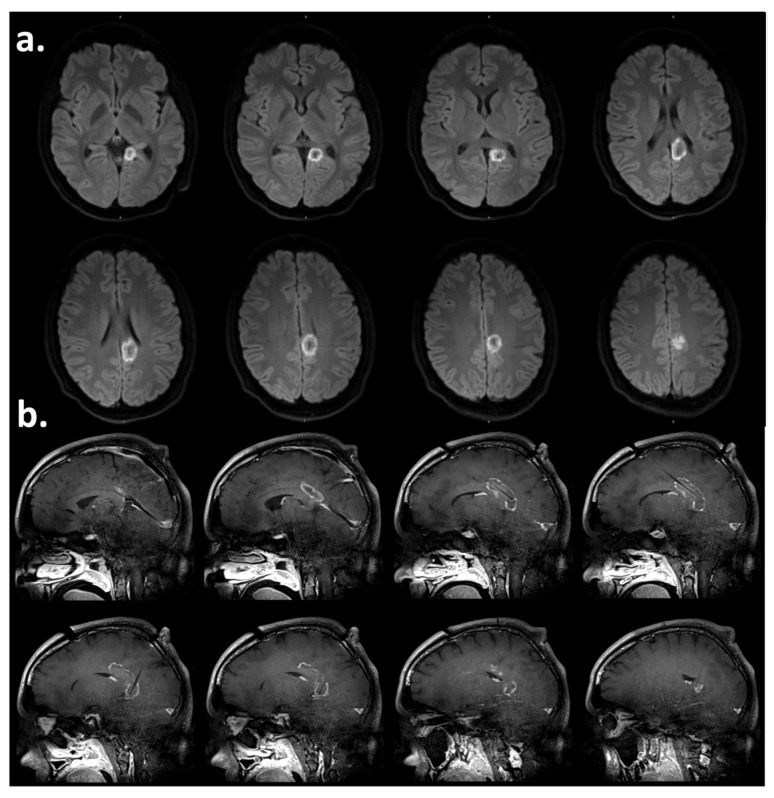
(a) Post-operative diffusion-weighted axial MRI and (b) T1-weighted contrast-enhanced sagittal magnetic resonance image showing the area of laser thermal ablation, including the posterior left cingulate gyrus and parietal isthmus, as well as a portion of the left forceps major.
